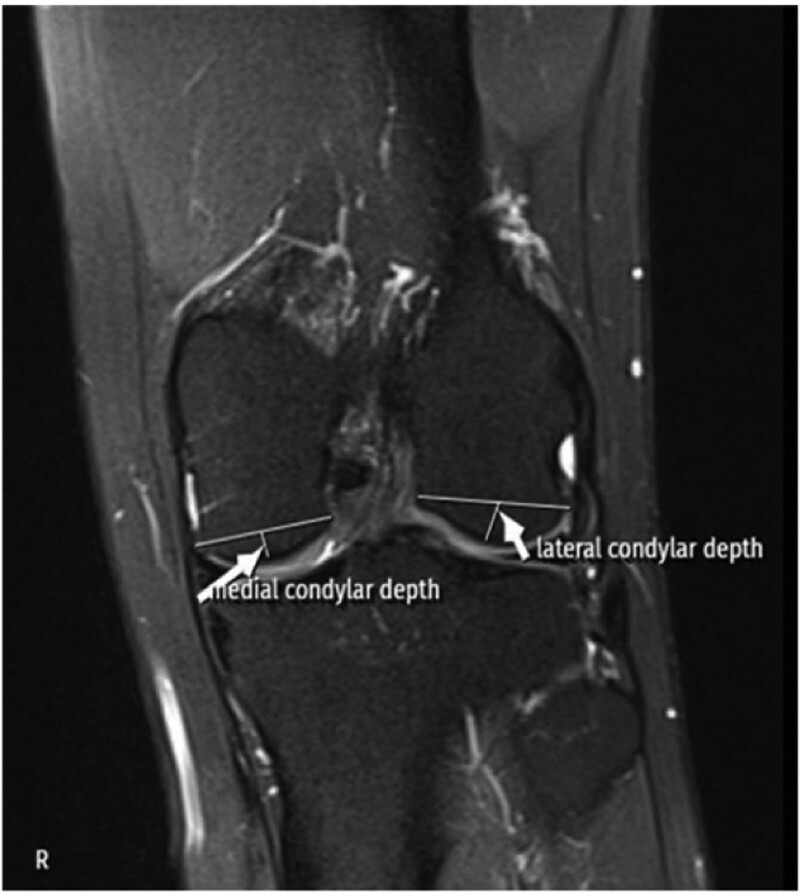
MRI measurement techniques of MFCD and LFCD. LFCD = lateral femoral condylar depth, MFCD = medial femoral condylar depth, MRI = magnetic resonance imaging.
